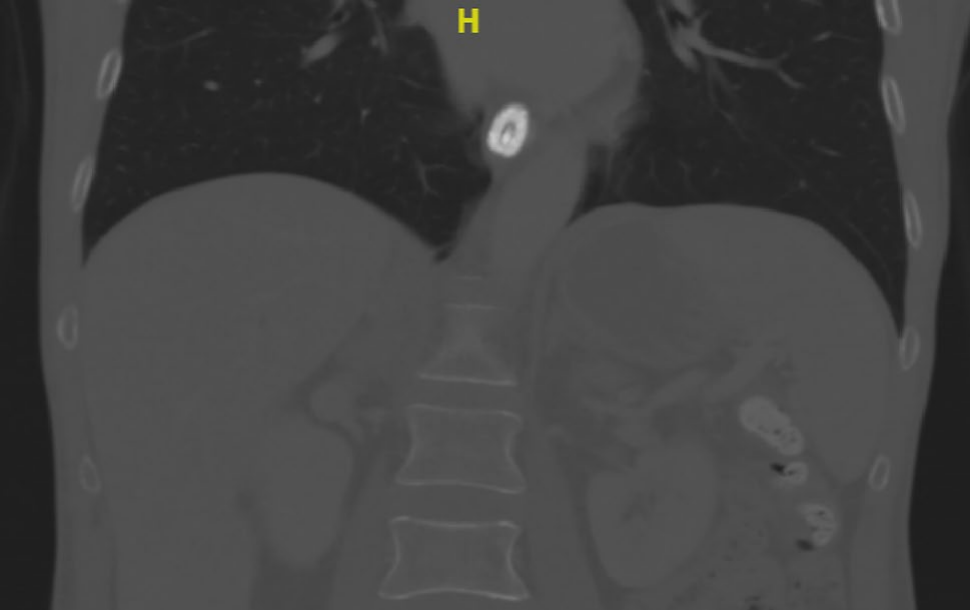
Coronal CT scan from patient 1 performed approximately 24 h after capsule endoscopy procedure showing the capsule stuck in the lower third of the esophagus
